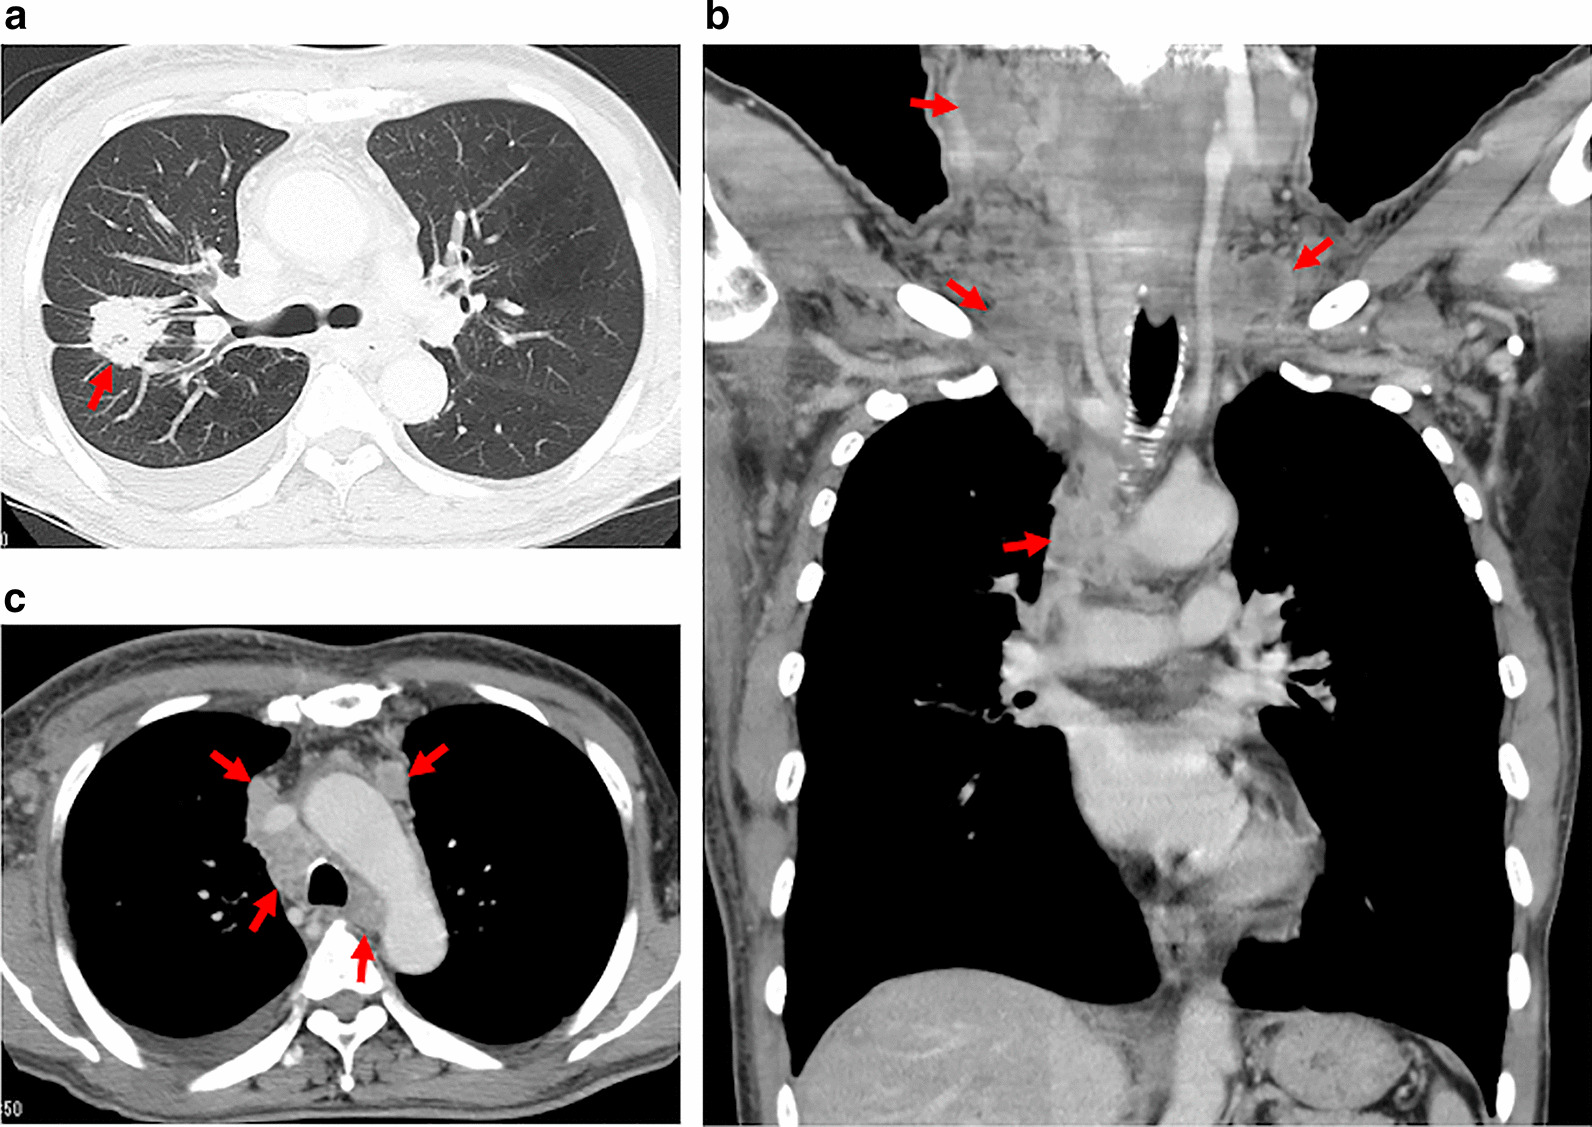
Chest computed tomography findings. a A tumor in the right upper lobe with hypertrophy of the bronchovascular bundles (arrow). b Lymphadenopathy of the hilar and mediastinal lymph nodes (arrows). c Lymphadenopathy of the mediastinal, supraclavicular, and cervical lymph nodes right dominantly (arrows)
